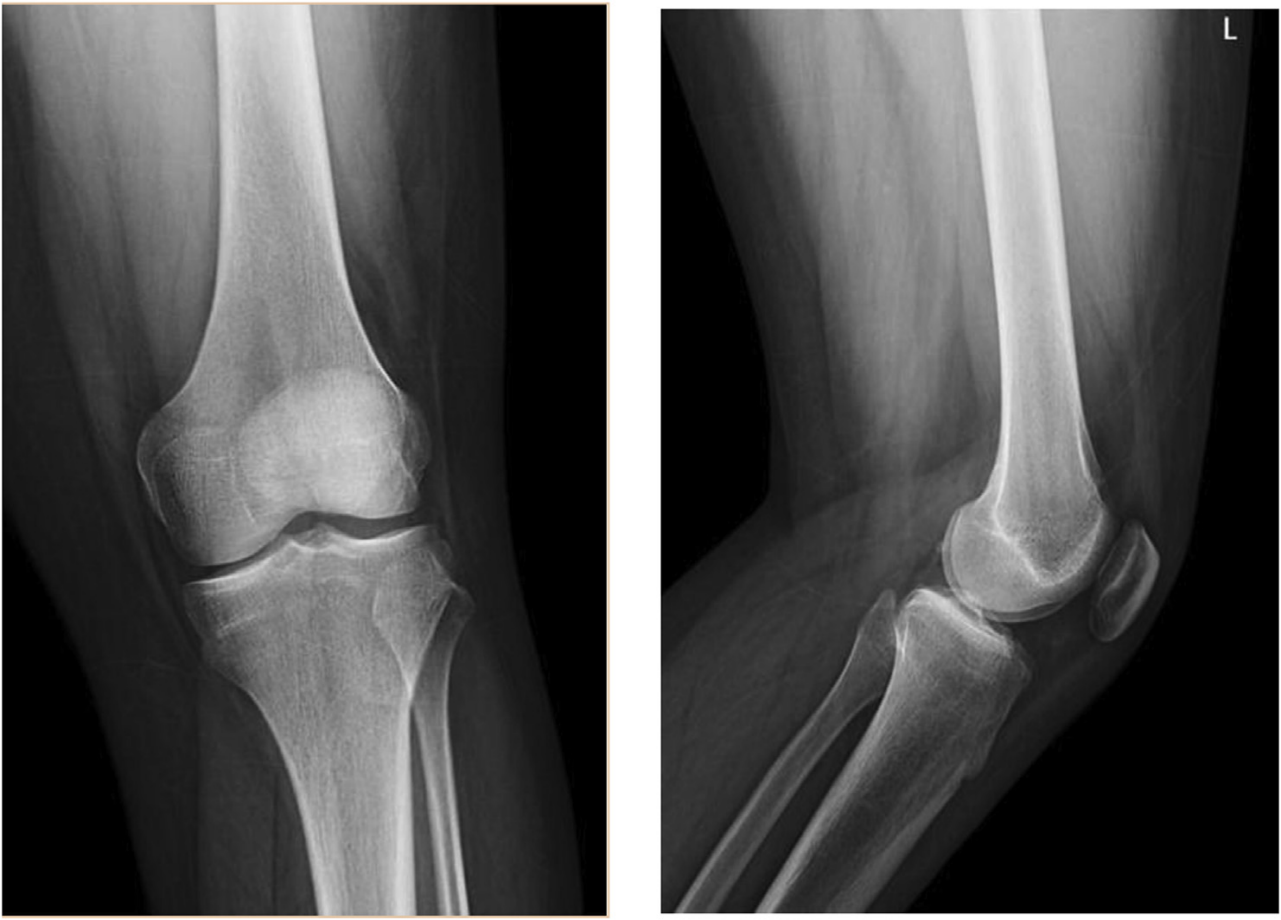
Anteroposterior and lateral x-ray views of left knee were reported as normal.
